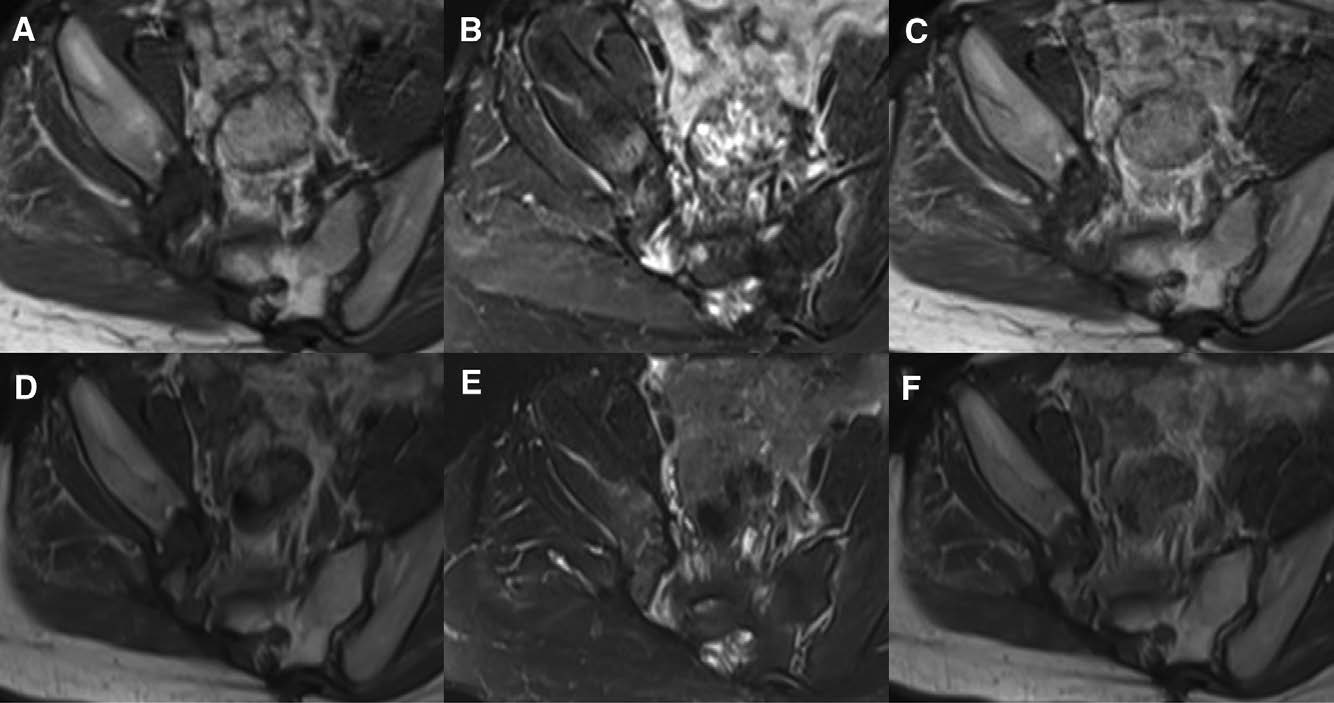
MRI acquired before and after imatinib treatment. (A and B) MRI showed a recurrence of the tumor on the right side of the pelvis, which demonstrated low intensity on T1-weighted images and mixed intensity on T2-weighted images dominated by high intensity. (C) MR contrast-enhanced showed heterogeneous contrast enhancement. (D and E) After treatment with imatinib, the lesion slightly shrank and exhibited low signal on T1-weighted images and slightly high signal on T2-weighted images. (F) MR contrast-enhanced showed heterogeneous contrast enhancement. MRI = magnetic resonance imaging.
